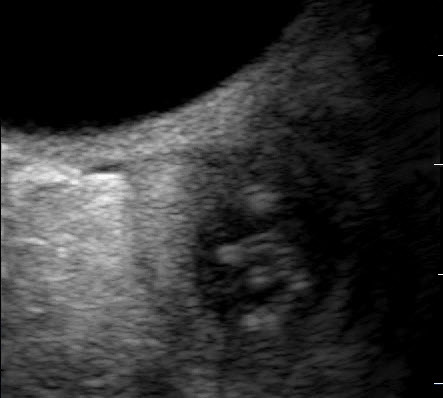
High-resolution ultrasound examination images showing a *Dirofilaria
